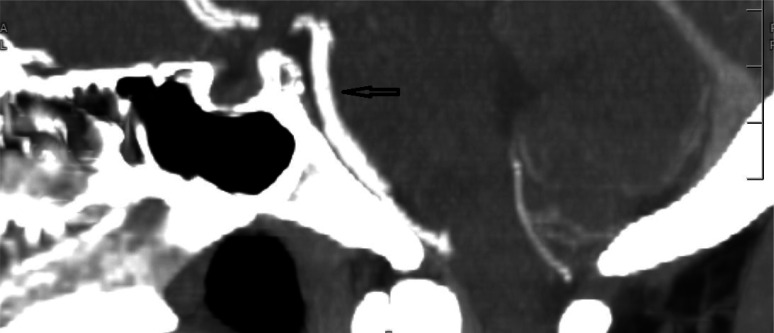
CTA sagittal section taken at 3-months post-diagnosis.
